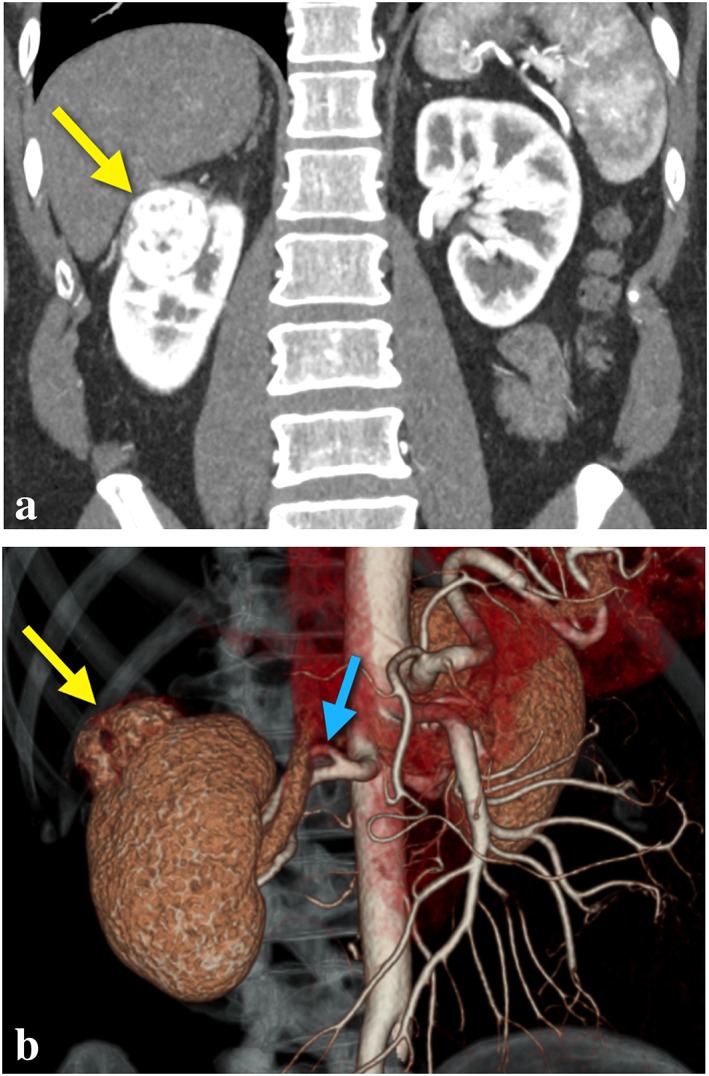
(a) Coronal CT scan demonstrating a predominantly endophytic right upper pole renal tumour (yellow arrow). (b) Same renal tumour (yellow arrow) with 3D reconstruction of CT renal arteriogram identifying upper pole branch of renal artery (blue arrow) suitable for selective occlusion RPN
